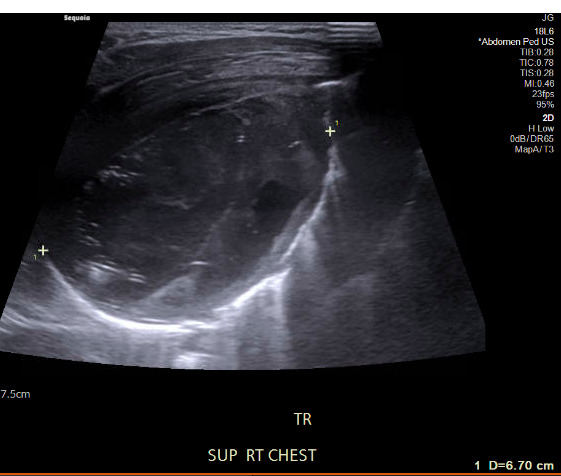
Radiology-based lung ultrasound demonstrating the lung lesion with similar measurements to the chest x-ray in the same encounter.
